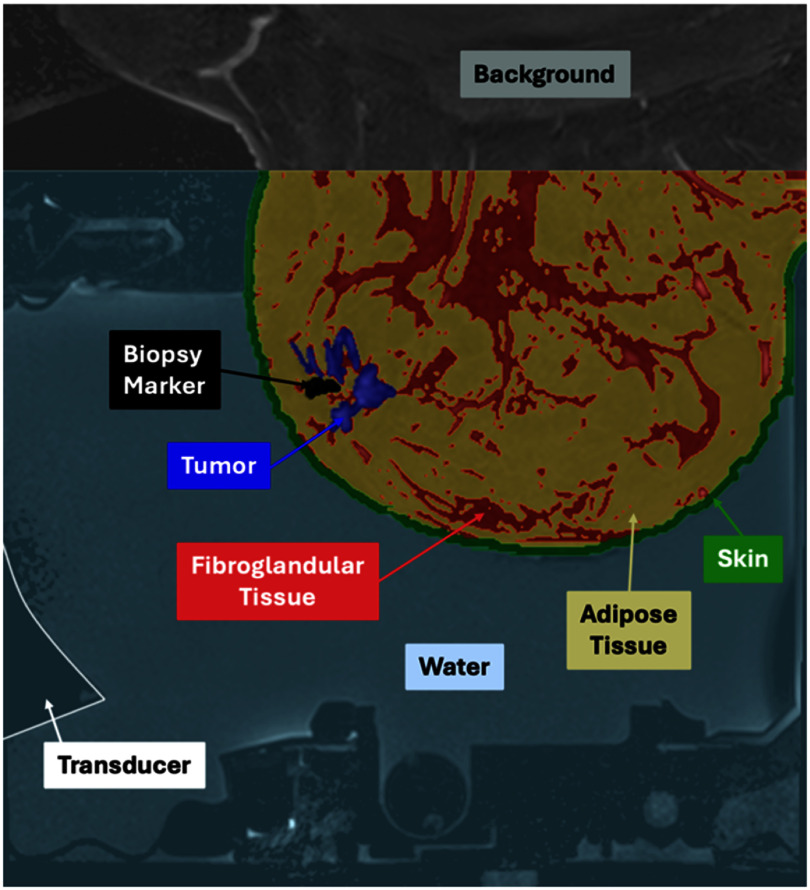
Breast segmentation example overlaid on a T1-weighted, dynamic contrast enhanced MRI of Patient 1.
